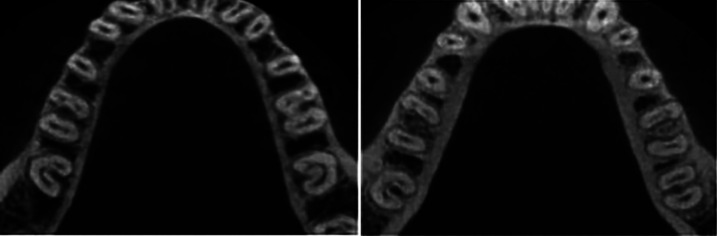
CBCT images of C-shaped molars. (a) Symmetrical and (b) Asymmetrical.
